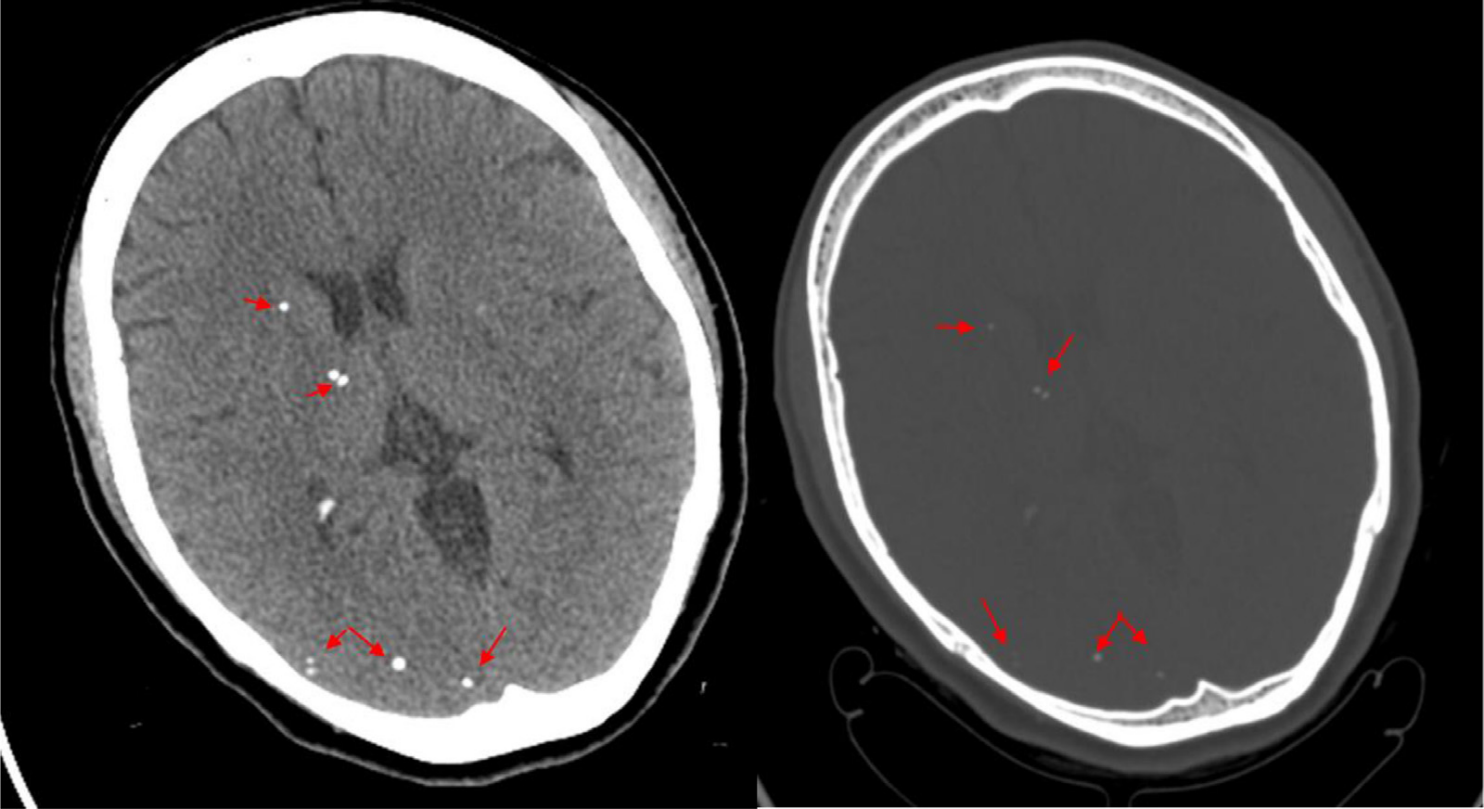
Axial CT of the brain with brain window (left) and bone window (right) showing multiple small cerebral calcifications [Red arrows], without focal mass or lesions. This can be seen in late stage neurocysticercosis or other infections such as toxoplasmosis. Differentials also includes metabolic abnormalities, such as hypoparathyroidism, or possible sequela of prior cerebral infarct.
